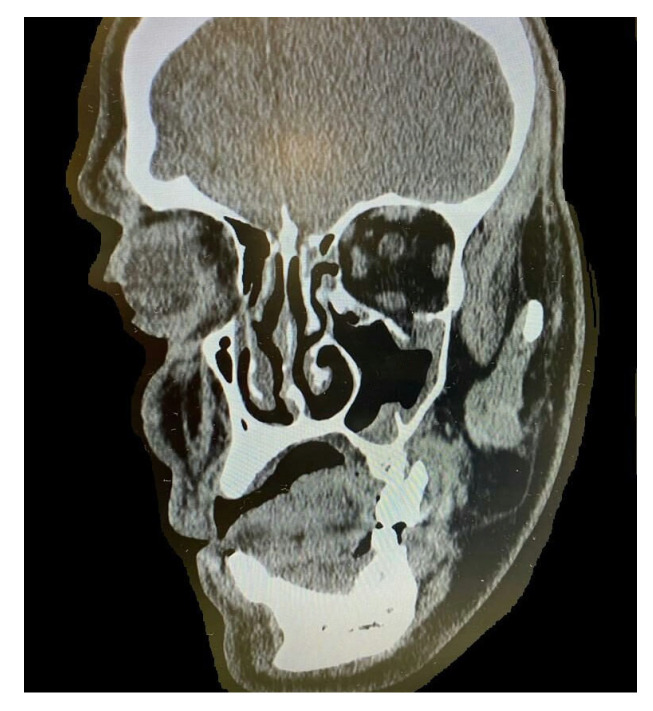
Cone-beam computed tomography (CBCT) of orbital floor fracture.
